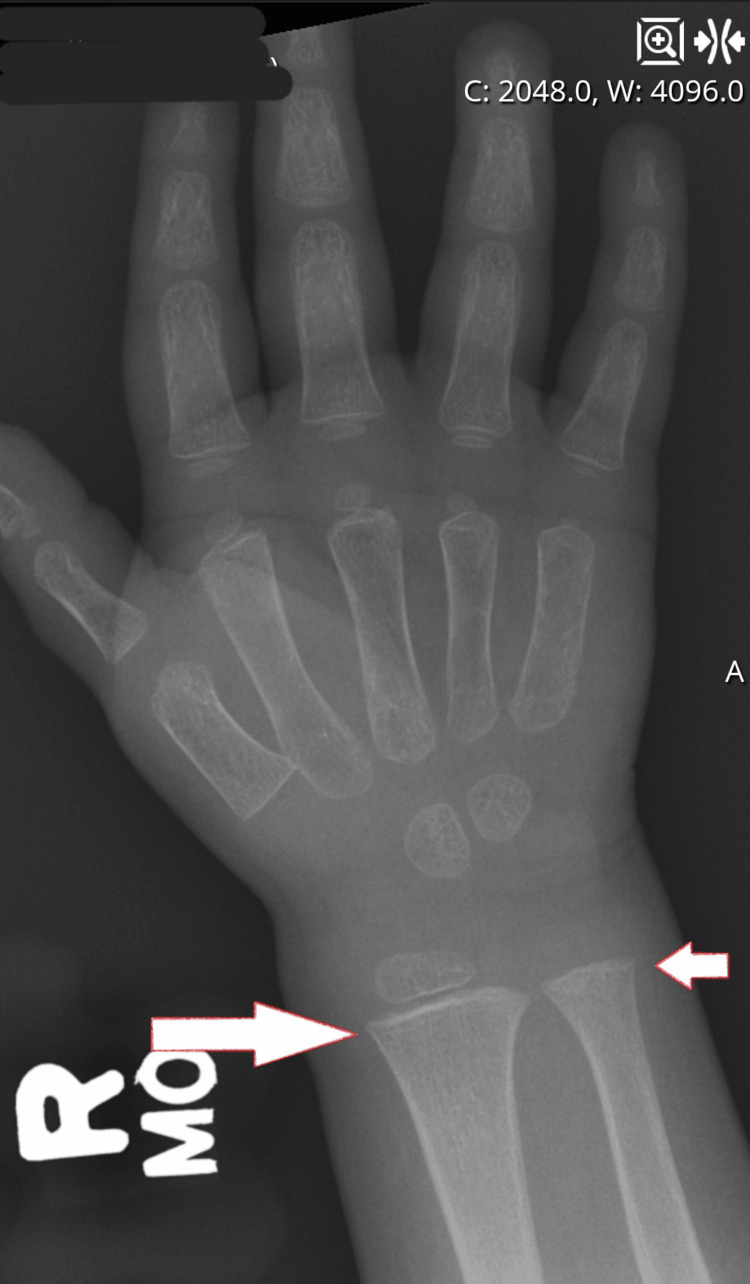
X-ray of the right wrist showing widened metaphases
